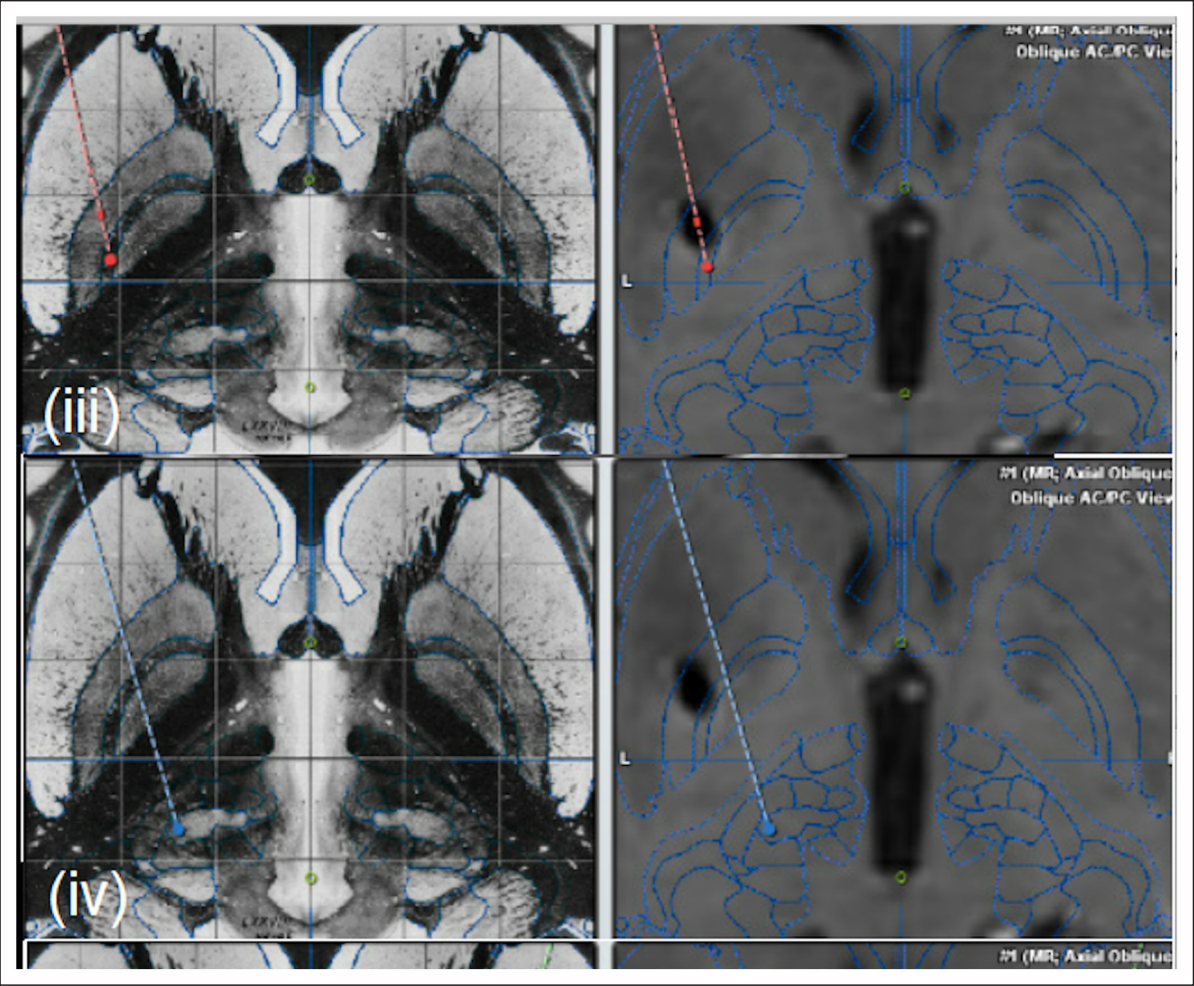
Localization of DBS electrodes. Electrodes locations were confirmed using BrainLab Stereotactic planning module. Following fusion of pre-operative MRI and post-operative CT, the electrodes were marked on the CT images. Atlas views were matched to the MRI images based on anatomy. (iii) Matched atlas view of the Lt. GPi lead. (iv) Matched atlas view of the Lt VIM lead.
